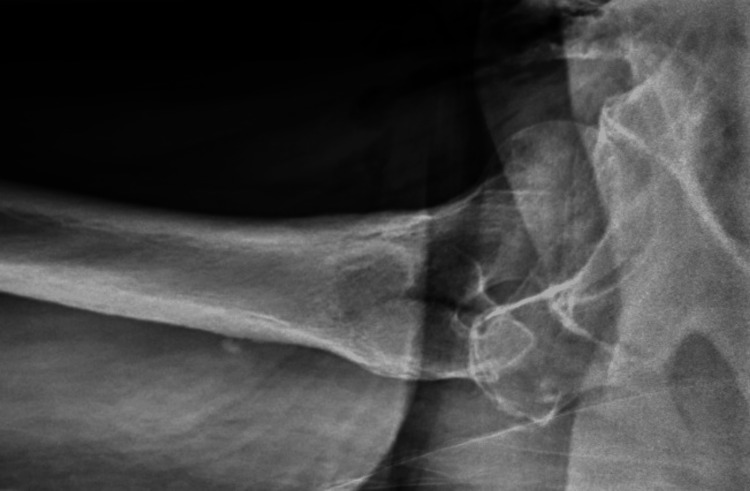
X-ray of the right hip showing no fracture, with surgical emphysema.
